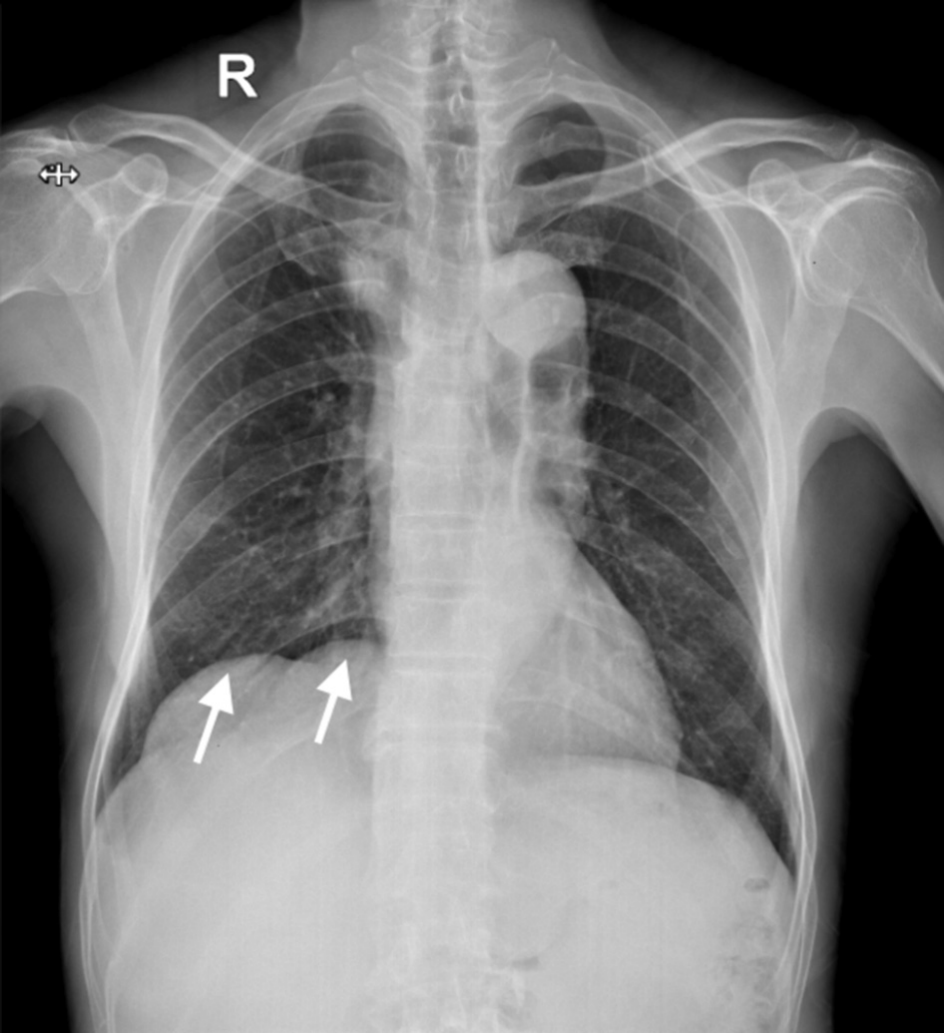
Two dome-shaped elevations of the right hemidiaphragm on the chest radiograph (*arrow*).
